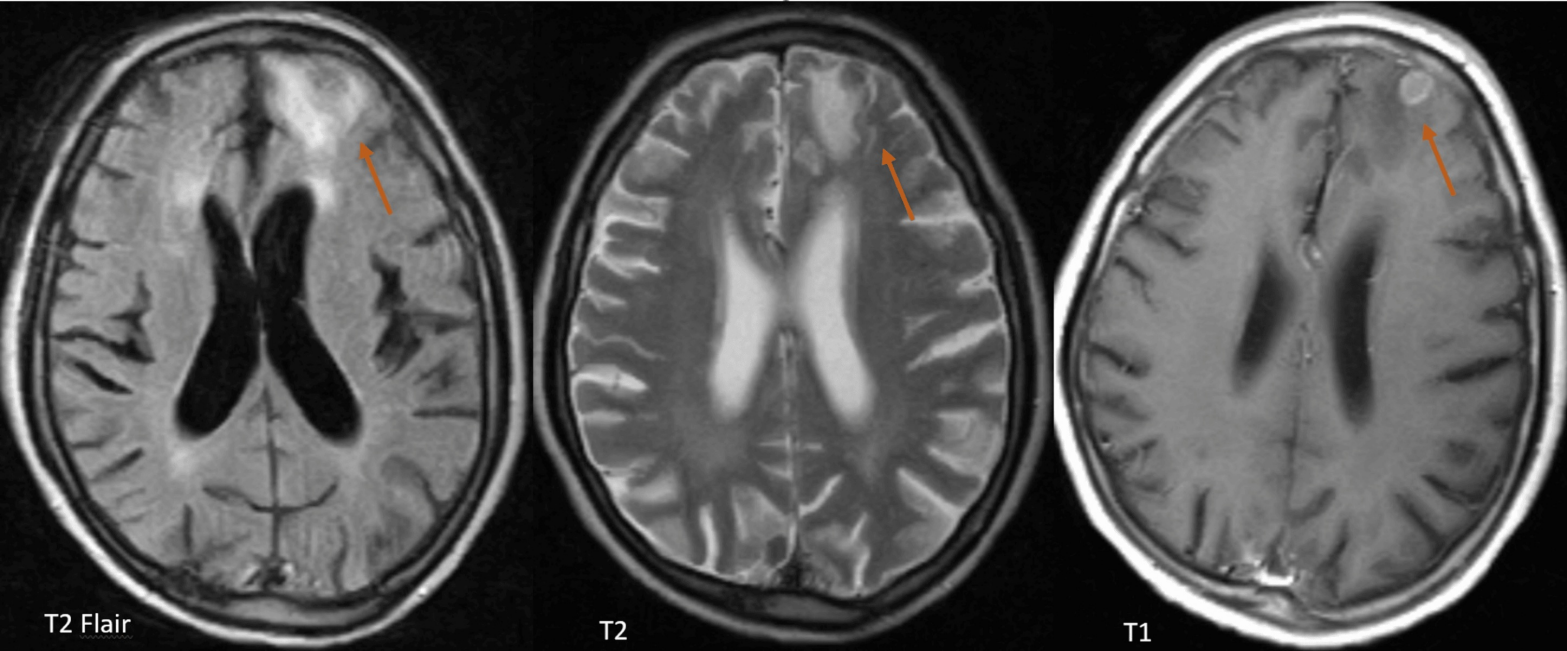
Magnetic resonance images of frontal neurocysticercosis lesions with perilesional oedema characteristic of the acute phase of the disease.
